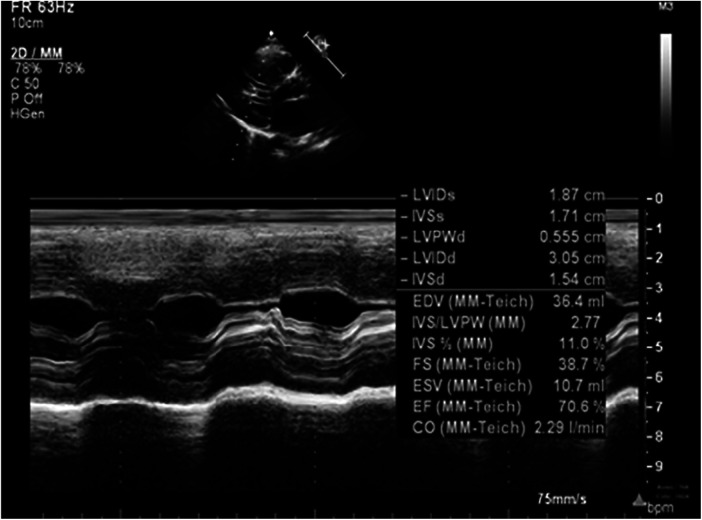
Echocardiography at 4 m.
